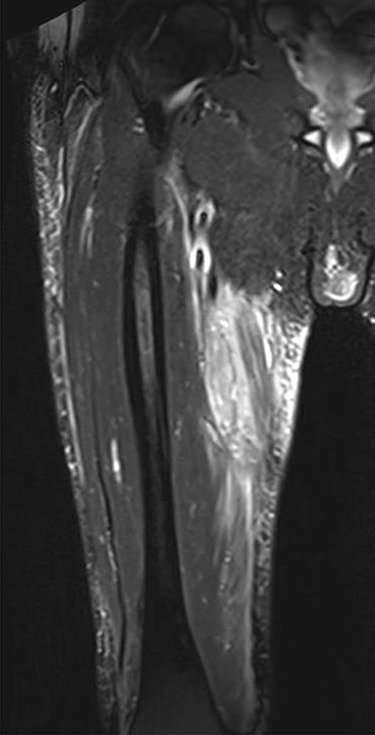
MRI sagittal view manifesting hematoma in right thigh region.
